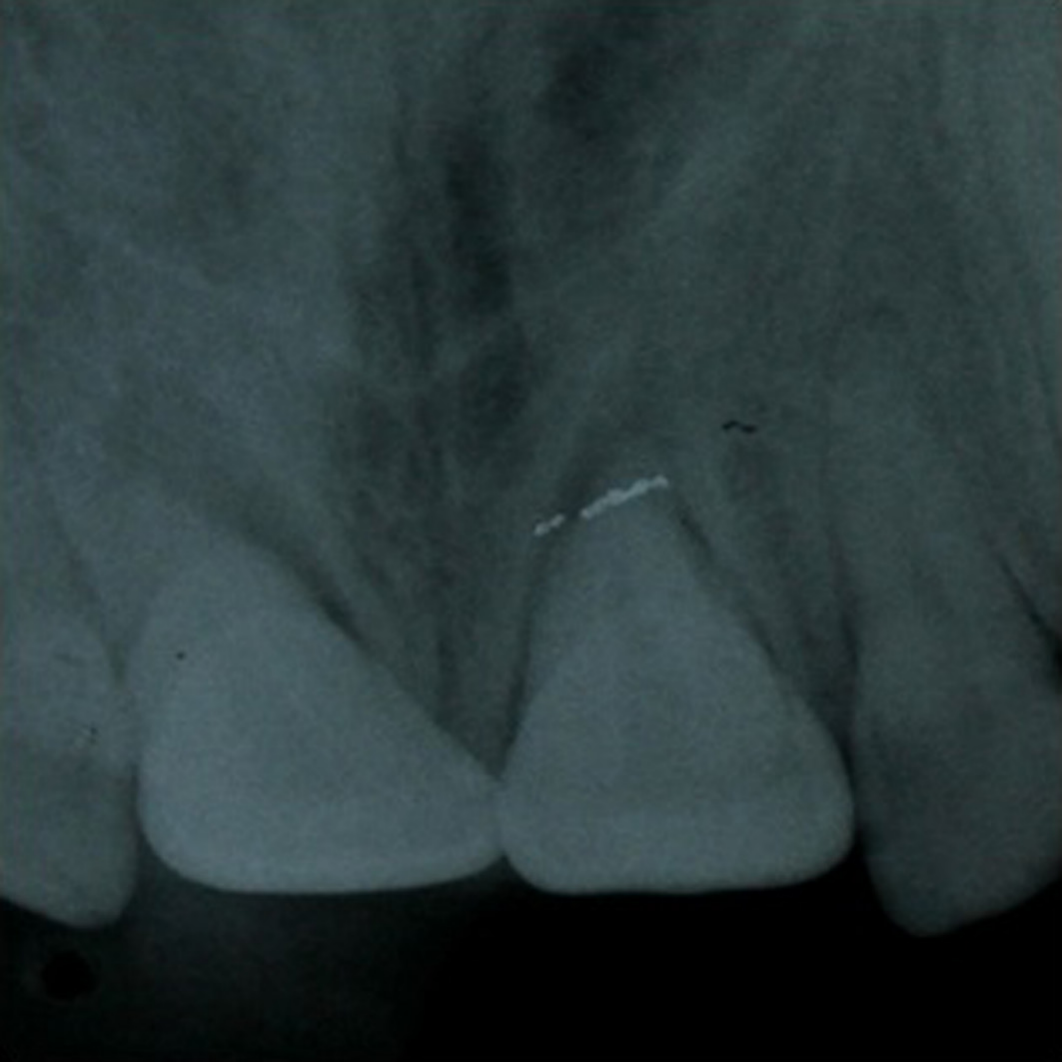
Initial periapical radiograph
